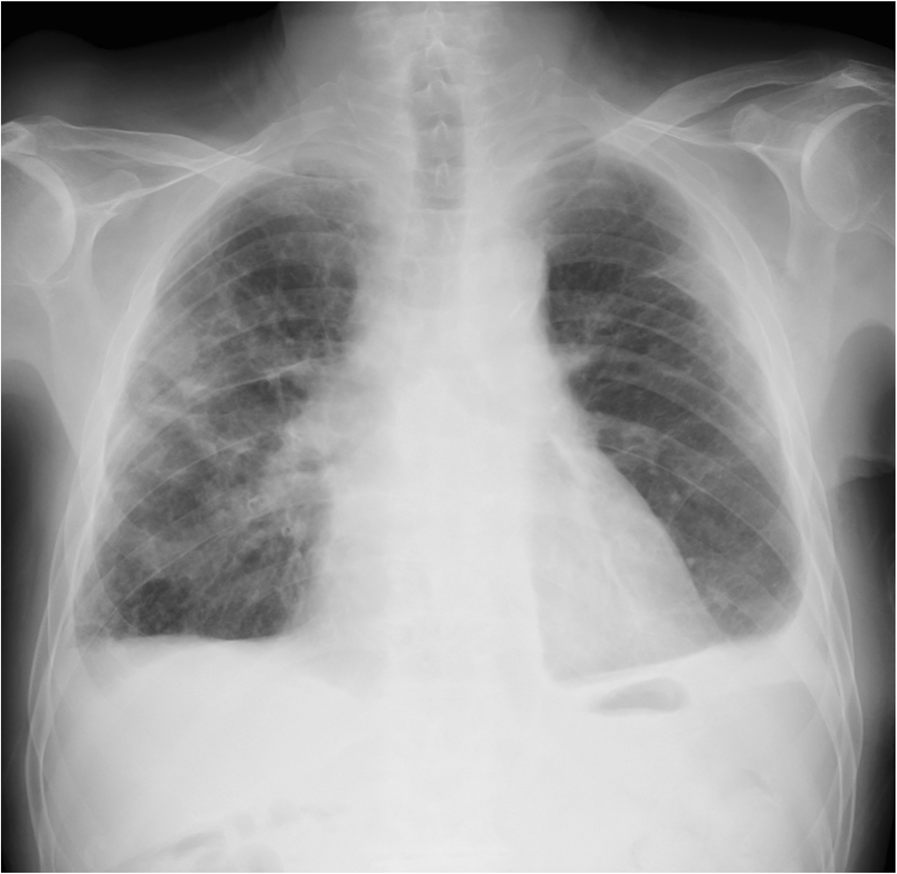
Postoperative chest radiograph showing full expansion of the lung
